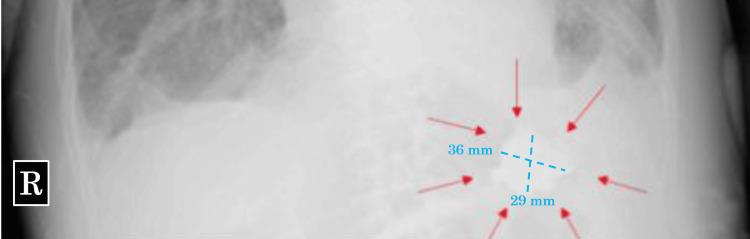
Chest X-ray on day 13 Chest X-ray shows persistent radiopaque material (red arrow) in the stomach.
